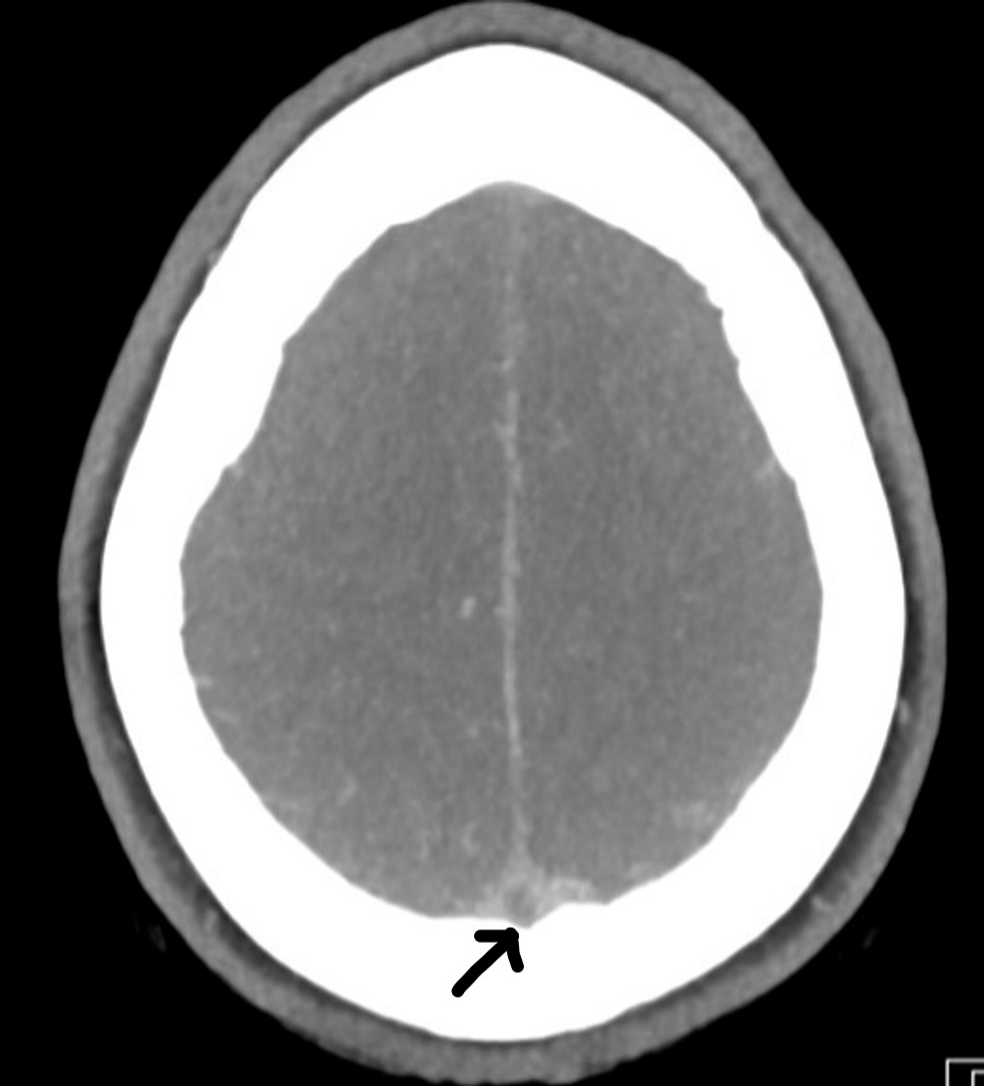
CT venogram showing reverse delta sign
